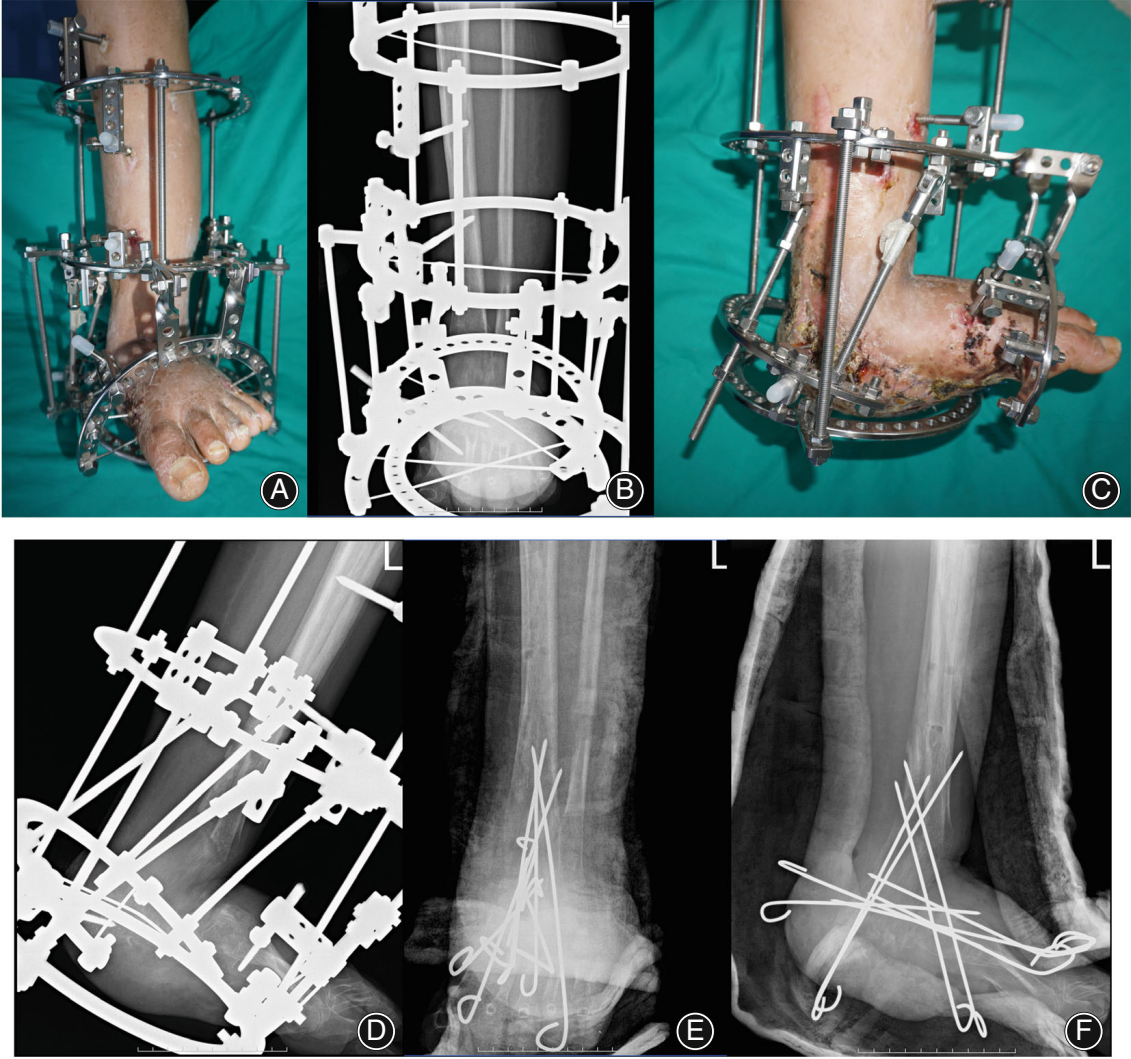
Appearance and radiography before and after external fixation was removed. Remnant of the calcaneus had been removed before the external fixation was applied. Supramalleolar osteotomy of the tibia and fibula was performed to distract the distal block toward the distal and posterior direction. (A, B) Anteroposterior view of the affected limb before the external fixation was removed. (C, D) Medial view of the affected limb before the external fixation was removed. Distraction osteogenesis was successful as the callus can be easily seen on radiographs. (E, F) Splint and Kirschner wires were applied after removal of the external fixation to enhance callus consolidation.
